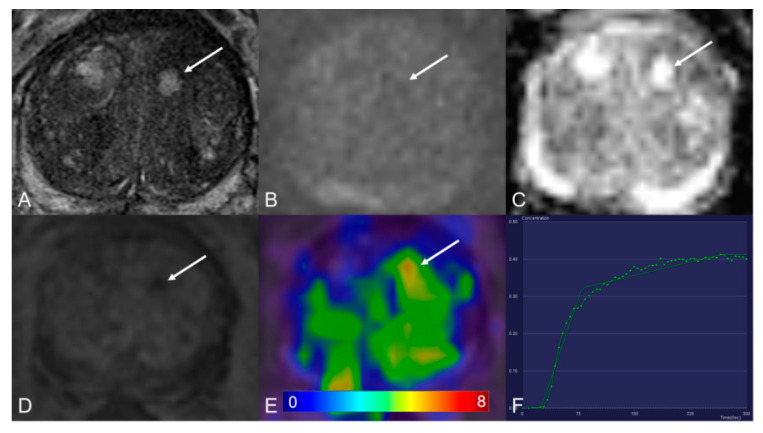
Transition zone with a PI-RADS 1 change. (A) Axial T2WI shows typical benign prostatic hyperplasia (BPH) change. (B) DWI (b = 1000 s/mm^2^) shows no lesion with a marked hyperintense signal above the background. (C) ADC map image presents no diffusion restriction. (D) Early dynamic contrast-enhanced image presents no enhancement within the typical BPH nodule. (E) [^68^Ga]Ga-PSMA-11 PET/MRI fusion image shows moderate [^68^Ga]Ga-PSMA-11 uptake, with SUVmax of 6.3. (F) DCE-MRI time-intensity curve demonstrates persistent increase enhancement. AT: 0.39 min; TTP: 1.09 min; W-in: 0.16; W-out: 0.02; PEI: 0.25; iAUC: 0.10.
